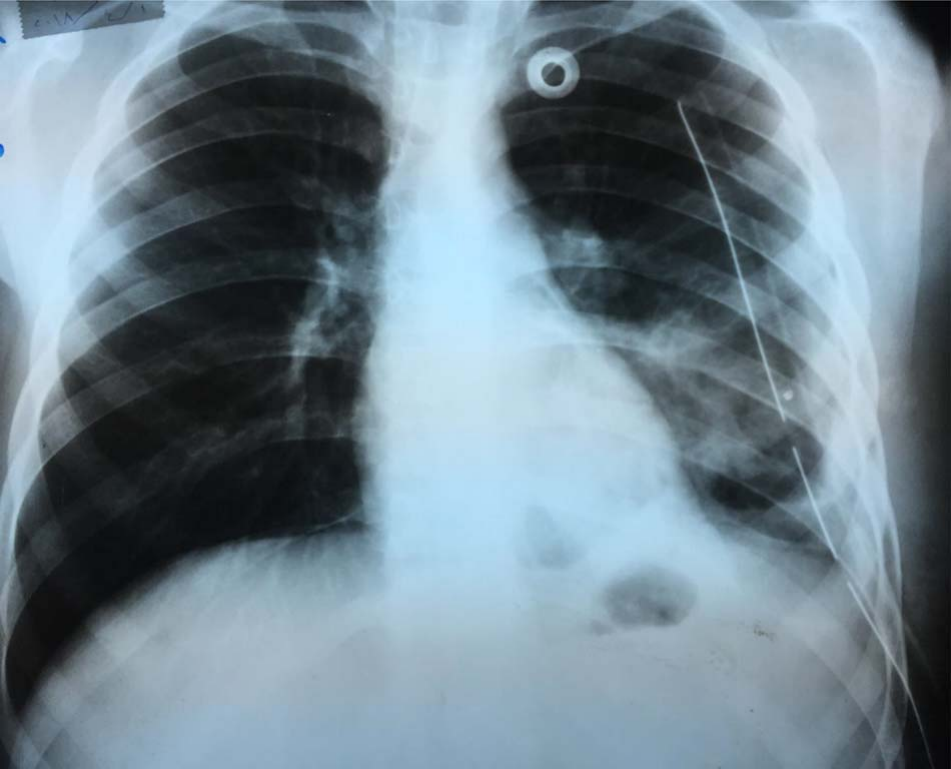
Chest X-ray 24 h after surgery.
